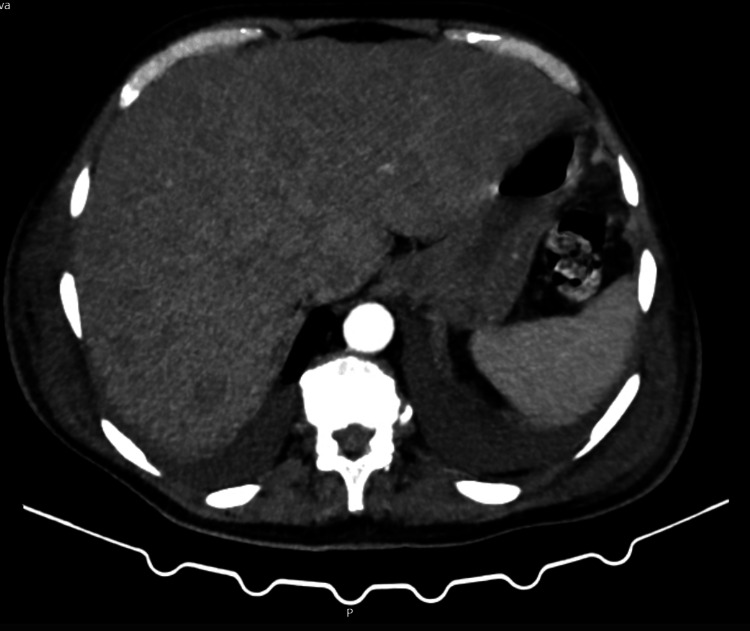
Thoraco-abdomino-pelvic CT revealed an enlarged liver with multiple nodular formations.
